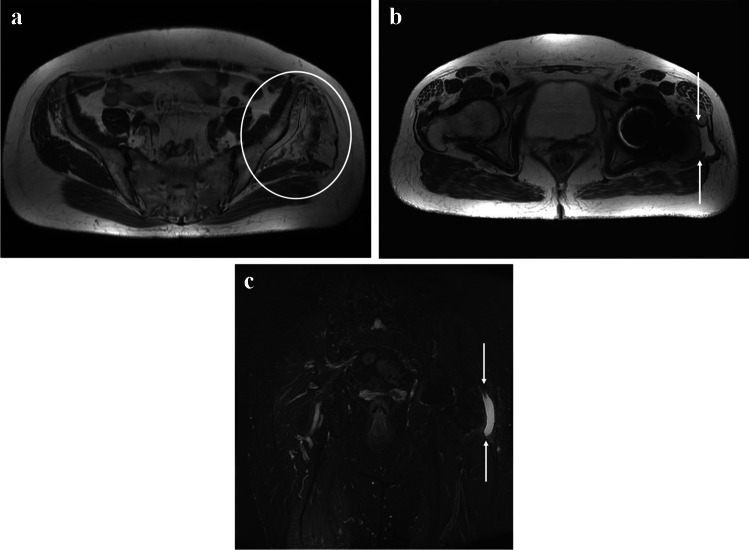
MRI showing left-side gluteus medius tendon tear at the level of insertion. Fatty atrophy on MRI a T1-weighted axial, b T2-weighted axial, and c STIR coronal
